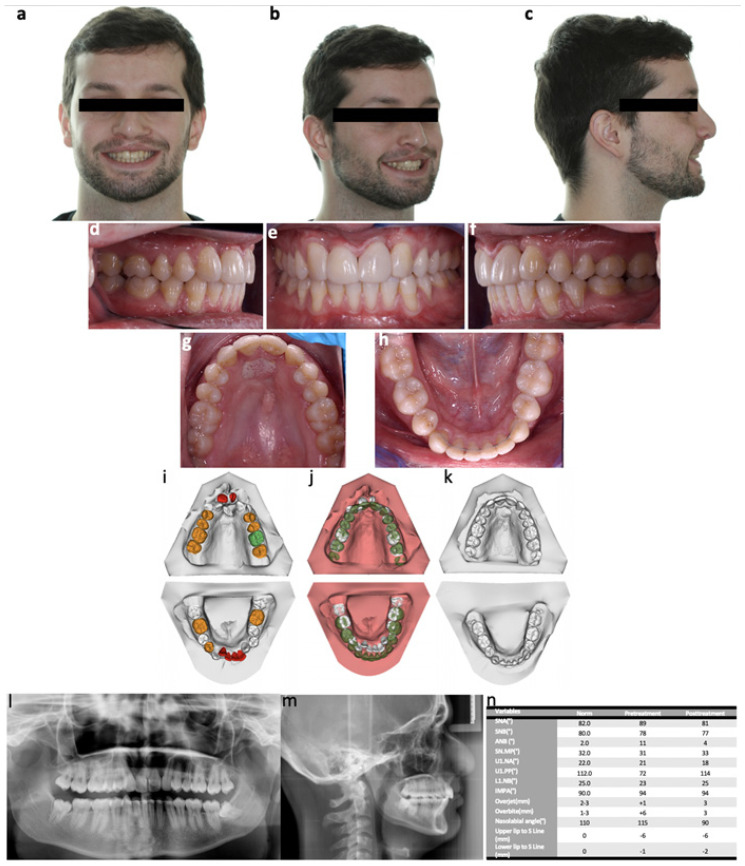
Post-treatment records: extraoral photographs (a) frontal view; (b) 3/4 lateral view; (c) profile; oral photographs (d) right side; (e) frontal; (f) left side; (g) superior arch; (h) inferior arch; dental casts (i) initial; (j) superimposition; (k) final; (l) final orthopantomography; (m) final lateral cephalogram; (n) cephalometric analysis.
